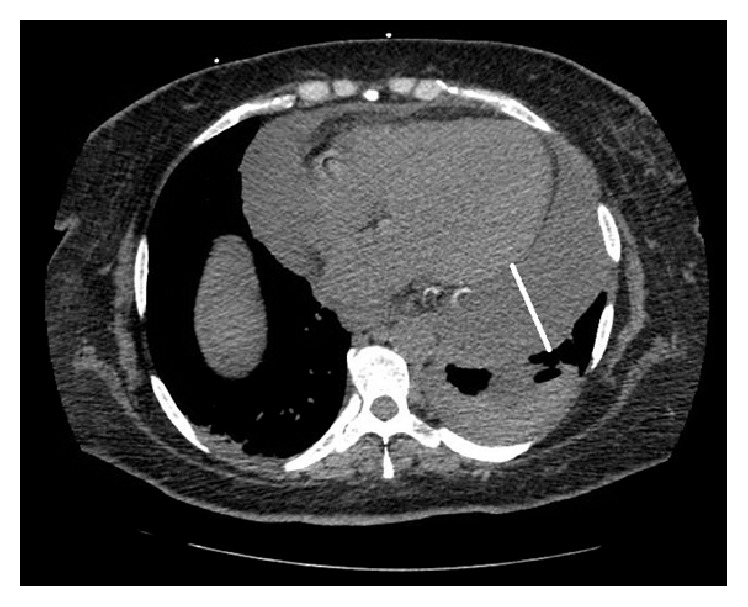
Axial CT chest showing a large pericardial effusion with a small left pleural effusion.
